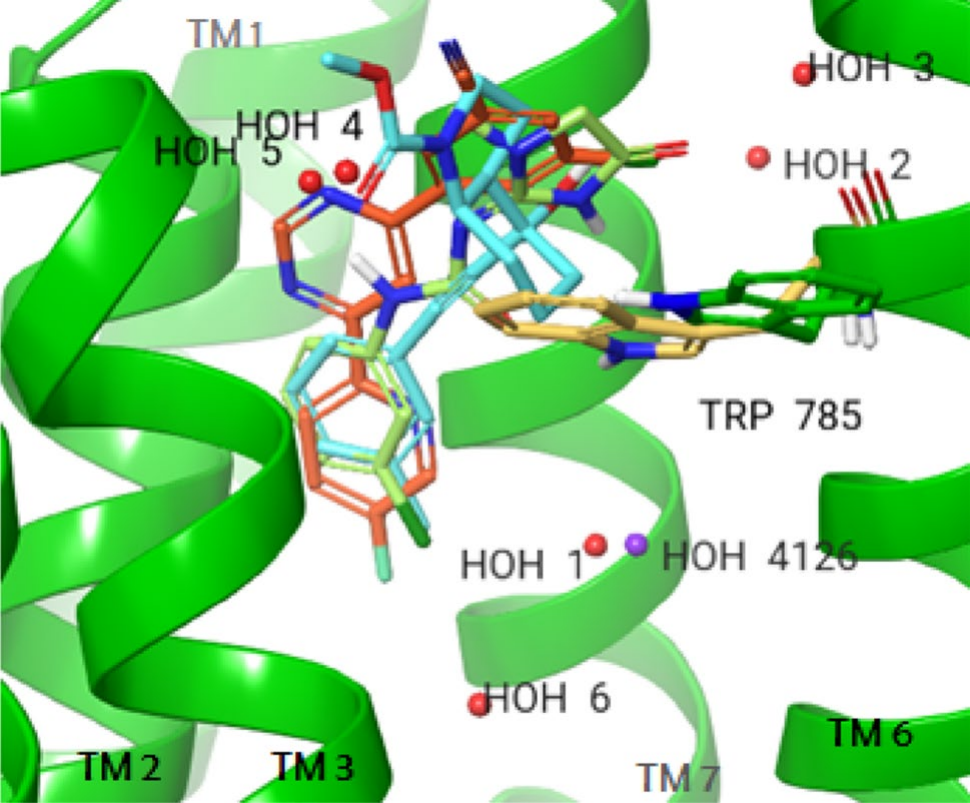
mGlu_5_ receptor binding pocket with mavoglurant (cyan), HTL-14242 (orange) and fenobam (light green). Water molecules generated by WaterFLAP (HOH1-6) and used in the docking studies are shown as red balls and X-ray water (HOH 4126) is shown as purple ball. Trp785^6.50c^ that adopts different conformation in the mavoglurant complex (shown as green sticks) as compared to the HTL-14242 and fenobam complexes (shown as yellow sticks) is also shown
